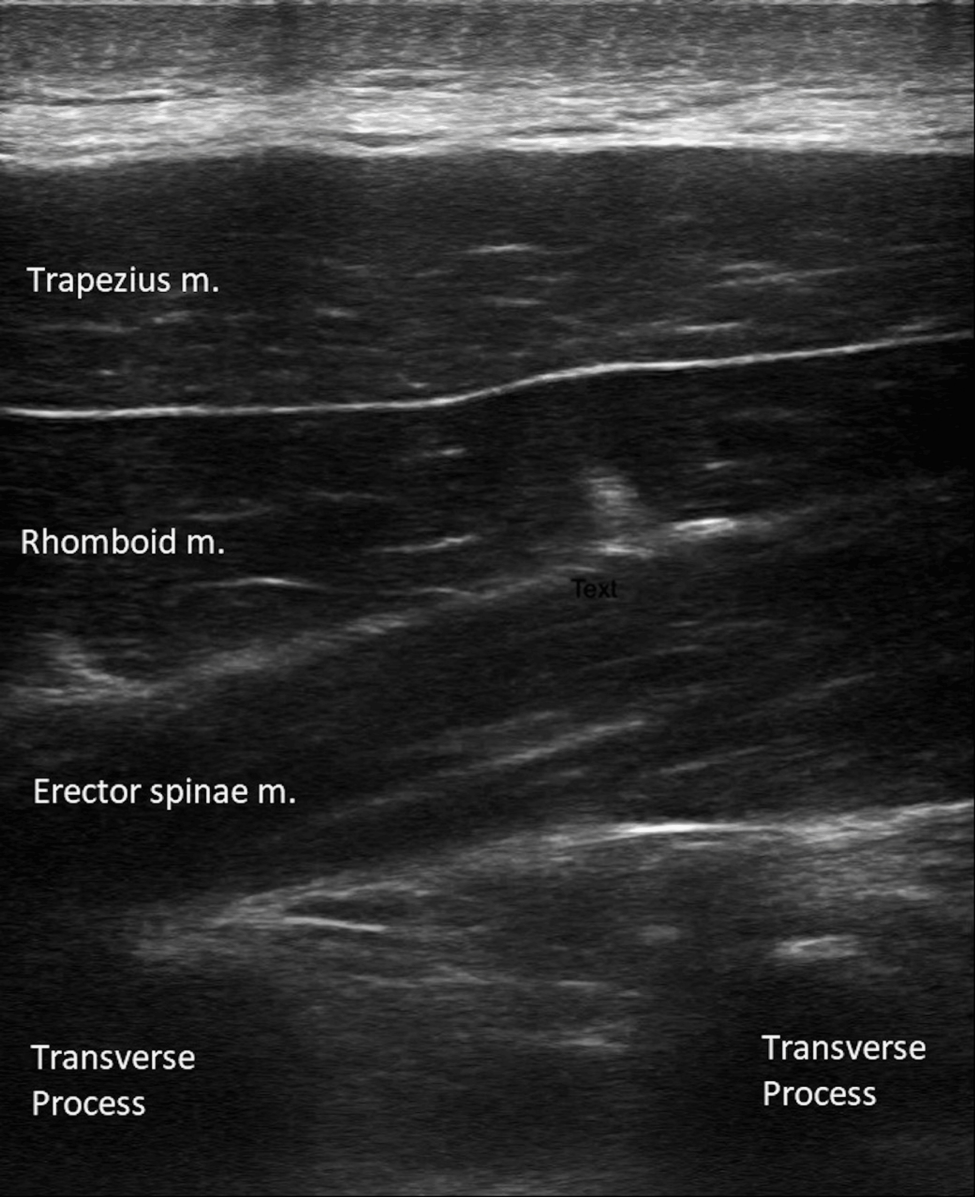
Ultrasound anatomy of the erector spinae plane block This is an original image produced by the authors of the paper.
